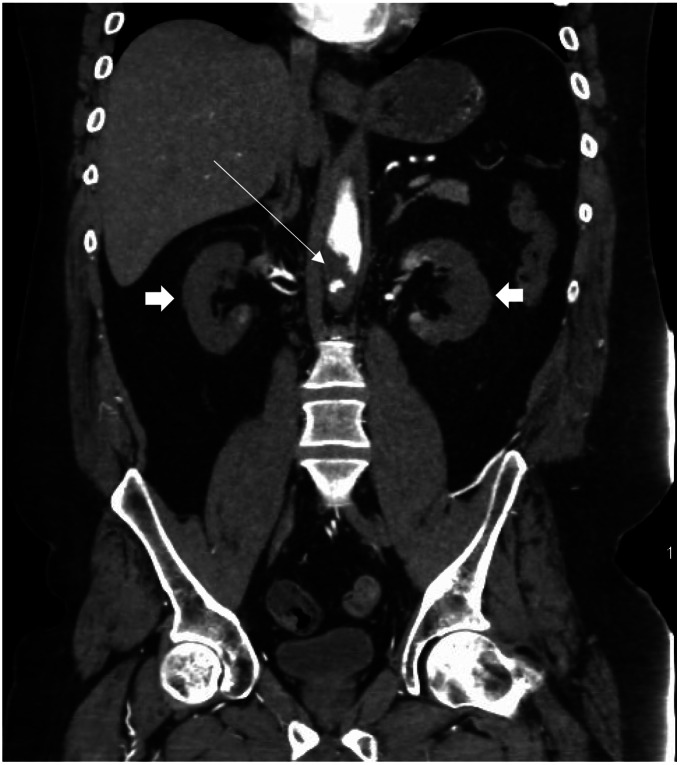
Computed tomography angiogram of abdomen in coronal plane showing aortic thrombus (thin arrow) and hypodense renal cortices (thick arrows).
